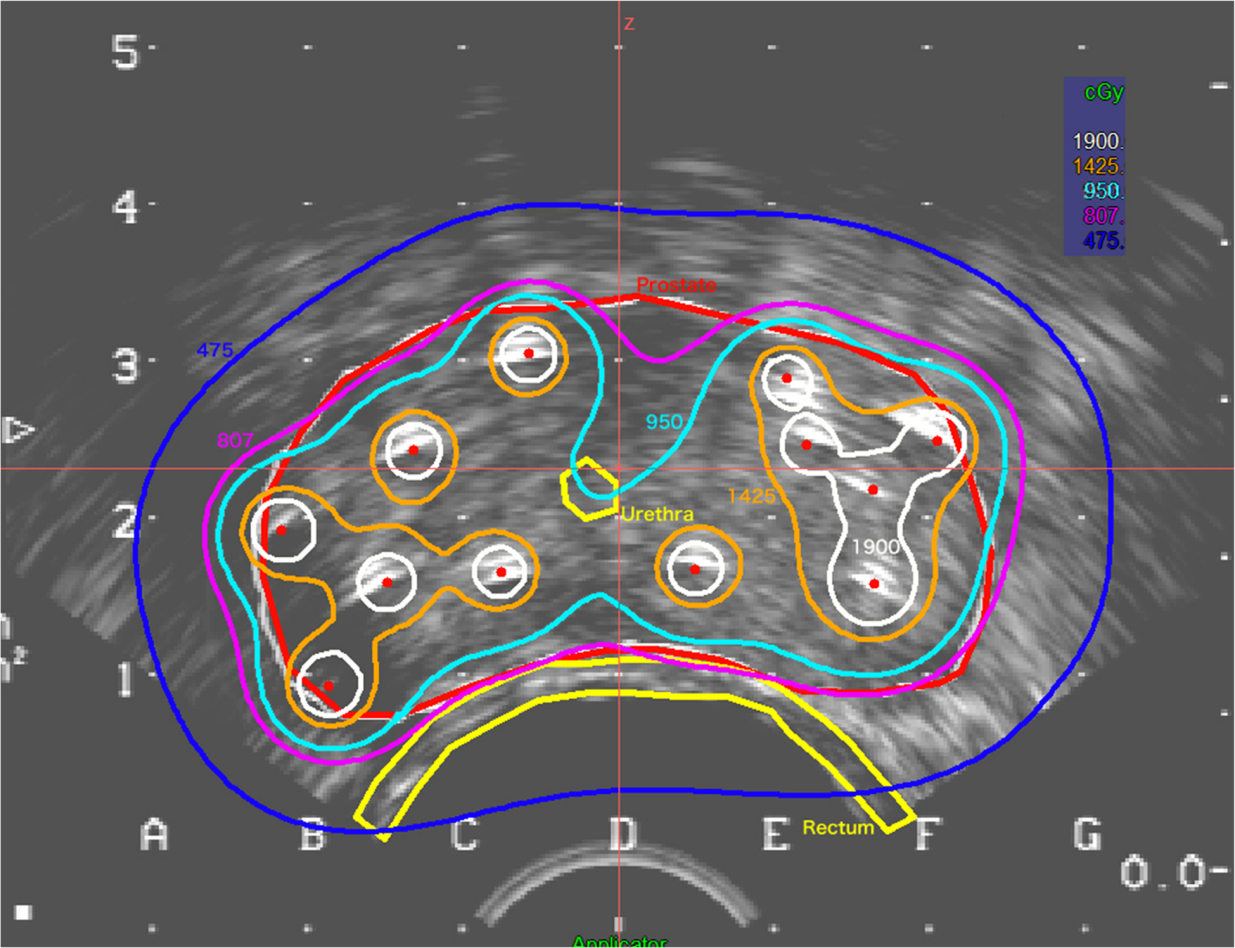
A typical ultrasound image of the applicator geometry used in HDR-BT. The red line represents the prostate or the planning target volume (PTV), respectively. Urethra and anterior rectal wall are depicted in yellow. The isodose lines are 50% = dark blue; 85% = purple; 100% = light blue; 150% = orange; 200% = white.
